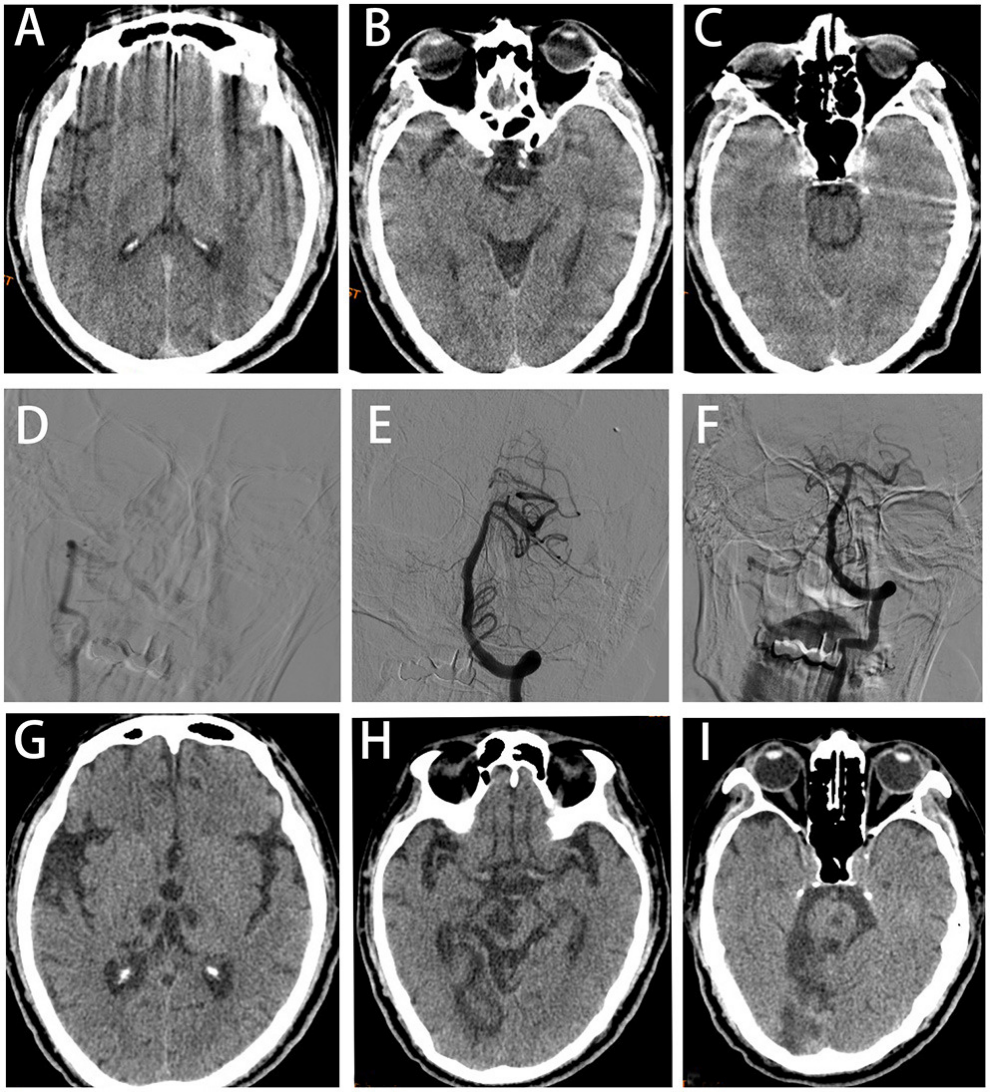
Case 2: (A–C) Axial brain computed tomography (CT) scan revealing no abnormalities. (D) AP right VA angiogram showing V4 segment occlusion. (E) AP left VA angiogram showing right superior cerebellar artery (SCA) and right posterior cerebral artery (PCA) occlusions; these vessels were successfully recanalized by endovascular thrombectomy (F). (G–I) Axial brain CT at 3 postoperative months showing low-density lesions in the bilateral thalamus, midbrain, right occipital lobe, cerebellar vermis and pons.
